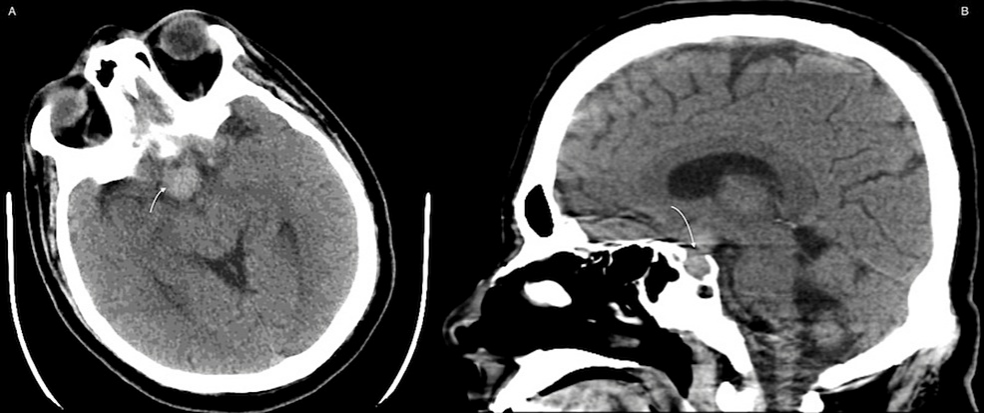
CT scan axial (A) and saggital (B) cuts, showing sellar hyperdensity representing the lesion of interest indicated by the white curved arrows in both cuts
